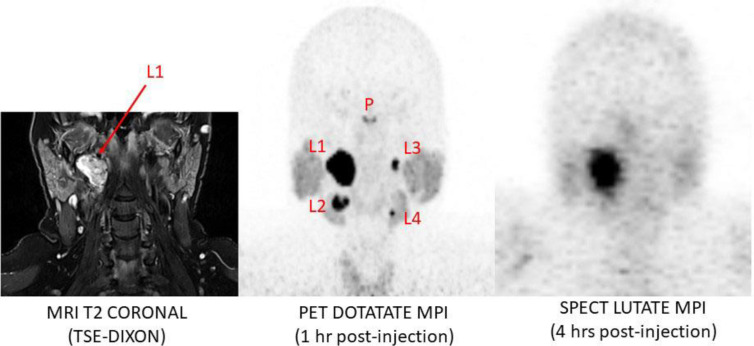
Comparative MRI, PET and SPECT imaging in a subject with somatostatin receptor positive multi-focal paragangliomas of the head and neck. The PET data were used to generate volumes of interest corresponding to the 4 lesions seen (L1 to L4) to examine the effect on dosimetry estimates. Dosimetry estimated by SPECT ranged from a 58% underestimate in the largest lesion (28 × 22 mm in maximal cross-section, 10.2 cc volume) to 99.5% underestimate in the smallest lesion (4 ×5 mm, 0.06 cc). See table 2 for estimates (P = Pituitary gland)
